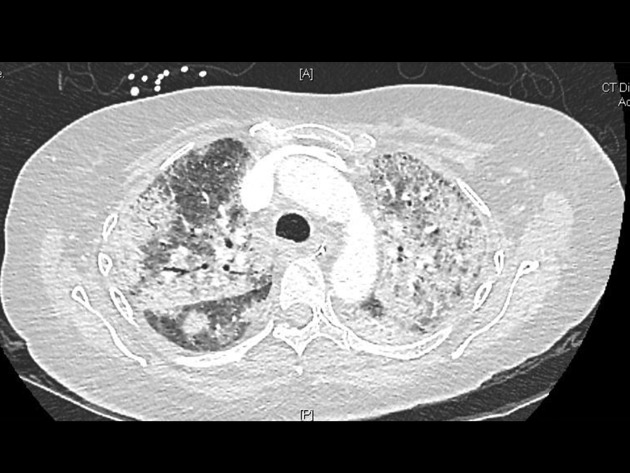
Thoracic computed tomography (CT) of the lungs showing diffuse ground glass opacities and bilateral consolidations in the upper pulmonary lobe.
